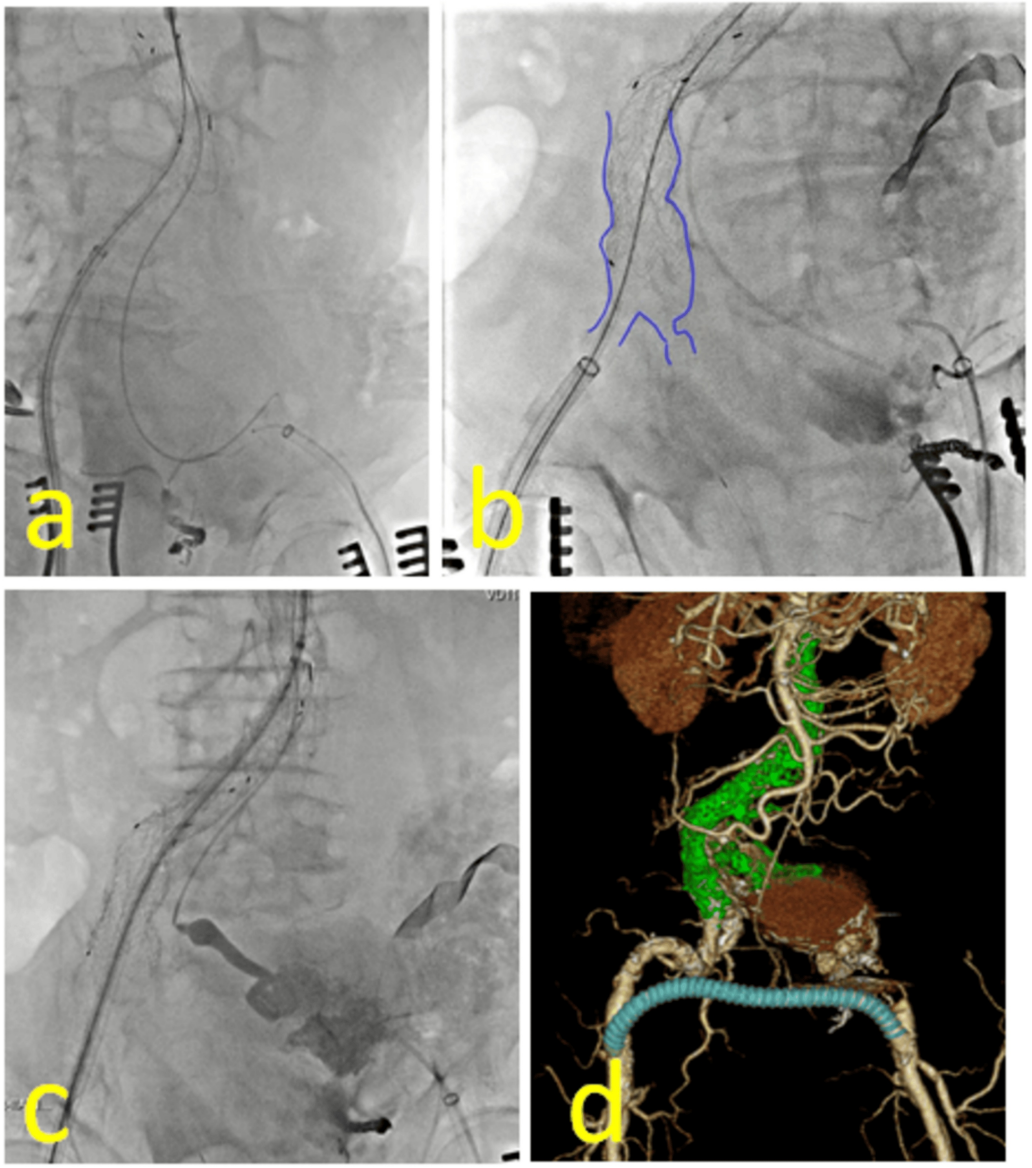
Intraoperative and postoperative findings (a) We advanced a pigtail catheter with a guidewire via the left brachial access to the left CIAA and attempted to catch the guidewire again in the CIAA via the left femoral access and to make a through-through, only to fail. (b) We delivered the upside-down stent graft via the right access using upside-down DrySeal® dilator whose distal edge was cut and deployed it in the trunk of the bifurcated Excluder® to occlude the contralateral opening. (c) The CIAA was embolized using n-butyl-2-cyanoacrylate via the left brachial access to control retroperitoneal bleeding. (d) Contrast-enhanced CT on POD 8 revealed a patency crossover bypass and no endoleak. CIAAs, common iliac artery aneurysms; CT, computed tomography; POD, postoperative day
